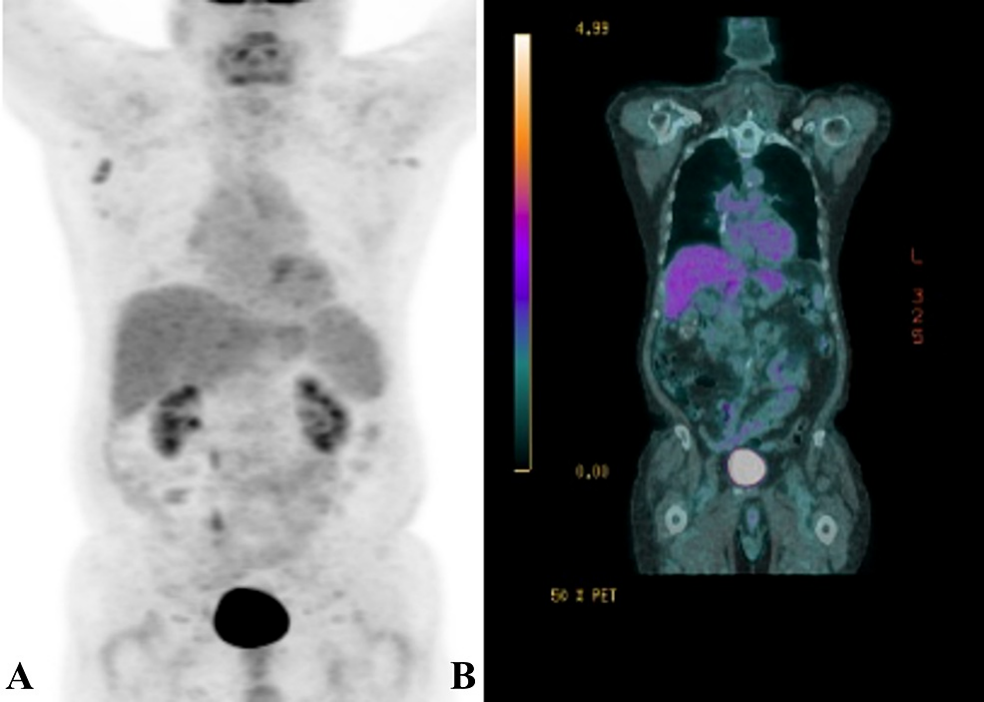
Repeat imaging following pembrolizumab therapy revealed complete radiological response Repeat imaging following two cycles of pembrolizumab ICI therapy using (A) positron emission tomography (PET) scan and (B) PET-computerized tomography (PET-CT) revealing complete radiologic response to therapy. ICI, Immune checkpoint inhibitor.
